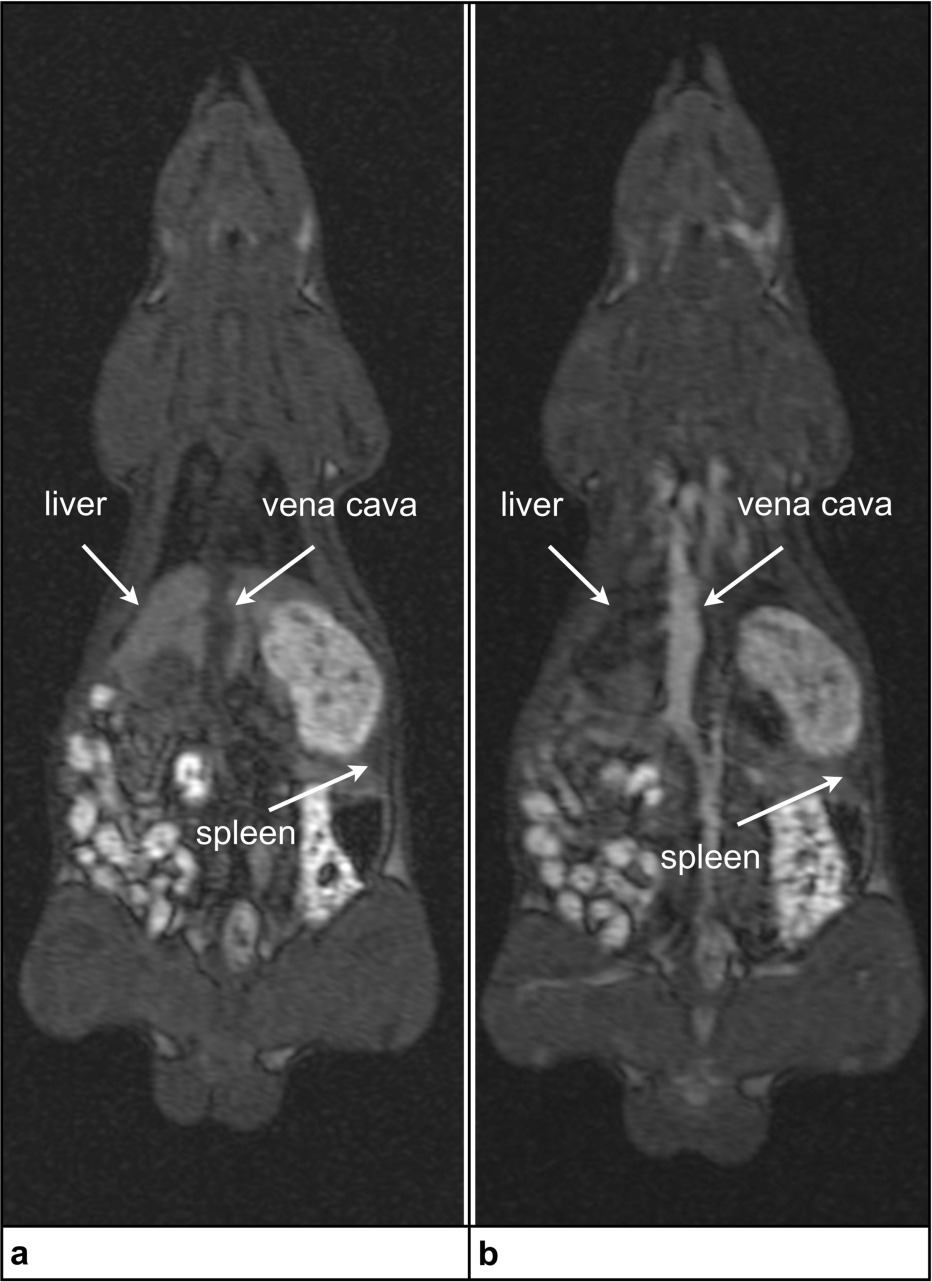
In vivo* MRI at 1.5 Tesla: T1-weighted 3D gradient-echo (GRE) fast low angle shot sequence. a–Image without administration of MCP 1; b–Image obtained 2 minutes after administration of 0.1 mmol Fe/kg of MCP 1.
